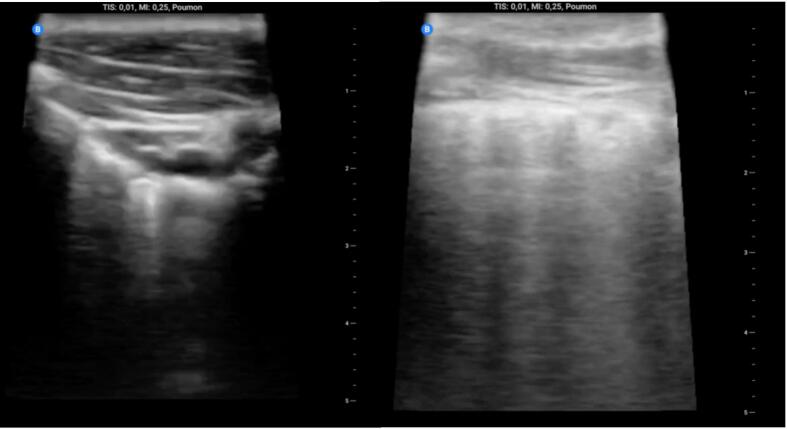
(INSERT VIDEOLINK): Left; sonographic image in lung mode at 5 cm (as a linear probe) depth of TB sequelae in the right apex. Note the broken irregular pleural line with loss of lung sliding and the lung pulse visible on B-mode due to massive destruction of the lung parenchyma. Right: sonographic image in lung mode (as a linear probe) at 5 cm depth of a miliary infiltrate extending to the lung periphery. Note almost regularly spaced B-lines with preserved lung sliding and the presence of granular subpleural hypoechoic consolidations.
